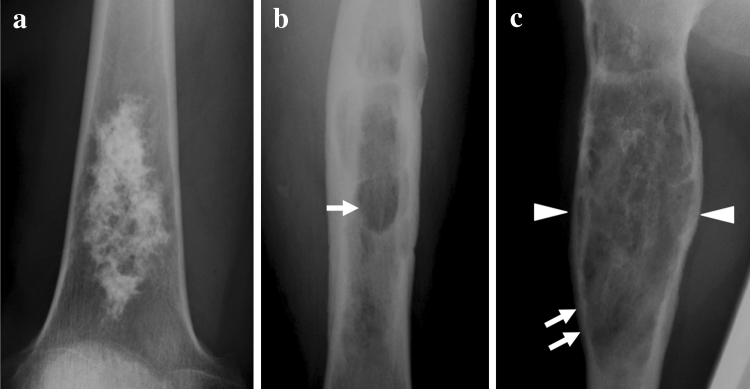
Radiographic findings of chondrosarcoma. a Presence of a ring-and-arc pattern of calcification. b Absence of calcification (*arrow*). c Presence of cortical penetration (*arrows*) and endosteal scalloping (*arrowheads*) is defined
